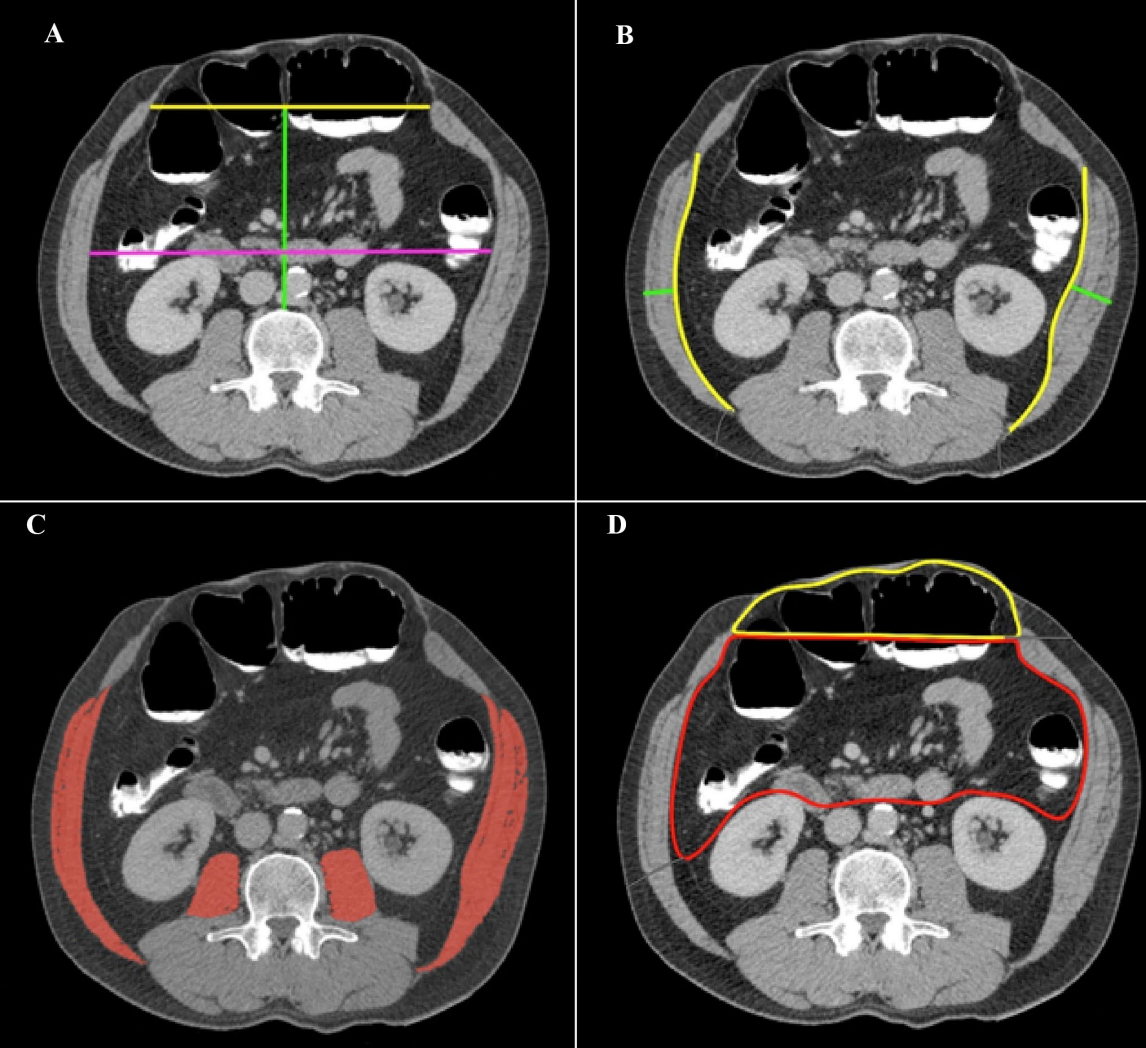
Measurements. A hernia width (yellow line), intra-abdominal width (purple line), and intra-abdominal depth (green line); B LAW muscle length (yellow line) and LAW muscle thickness (green line); C LAW muscle mass and density, and psoas muscle mass and density; D The intra-abdominal volumes and hernia volumes were obtained by encircling the intra-abdominal (red) and hernia (yellow) surfaces on all axial CT slides from the diaphragm to the pelvis. Then, the segmentation tool calculated the volumes. Loss of domain was calculated according to the Sabbagh method (hernia volume/(hernia- + abdominal volume)). *LAW* lateral abdominal wall
